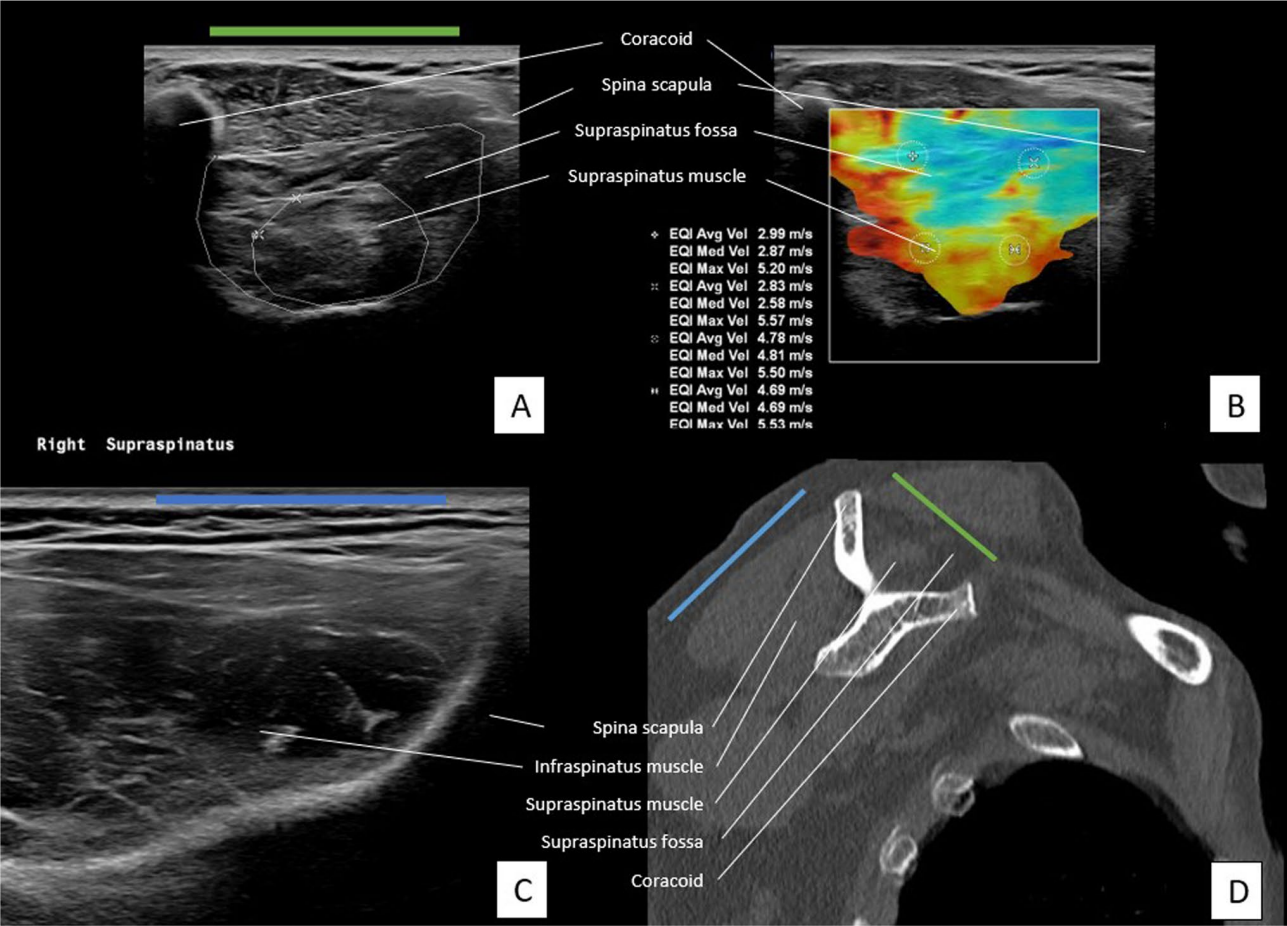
Assessment of SSP and ISP muscle on US and CT of patient severe muscle atrophy and fatty infiltration of the SSP muscle. A US plane of SSP. B US plane of SSP with measurements of SWV in m/s in four quadrants. C US plane of ISP. D oblique-sagittal plane of CT image to assess SSP and ISP muscle
